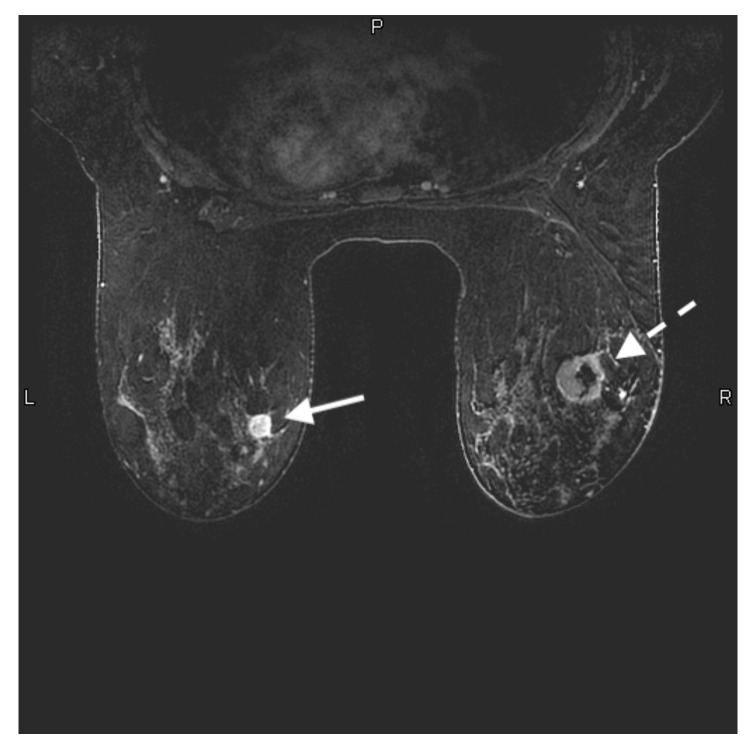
28-year-old woman with a known *BRCA* 2 genetic mutation presented for her baseline annual high-risk screening MRI. Axial contrast-enhanced T1-weighted fat-suppressed image shows a 1.4 × 1.3 × 1.2 cm rim enhancing mass in the upper inner left breast (solid white arrow). This was a biopsy-proven grade 3 invasive ductal carcinoma. There is also a 3.1 × 3.6 × 2.1 cm rim enhancing mass with central necrosis in the upper outer right breast (white dashed arrow). This was also biopsy-proven grade 3 invasive ductal carcinoma.
